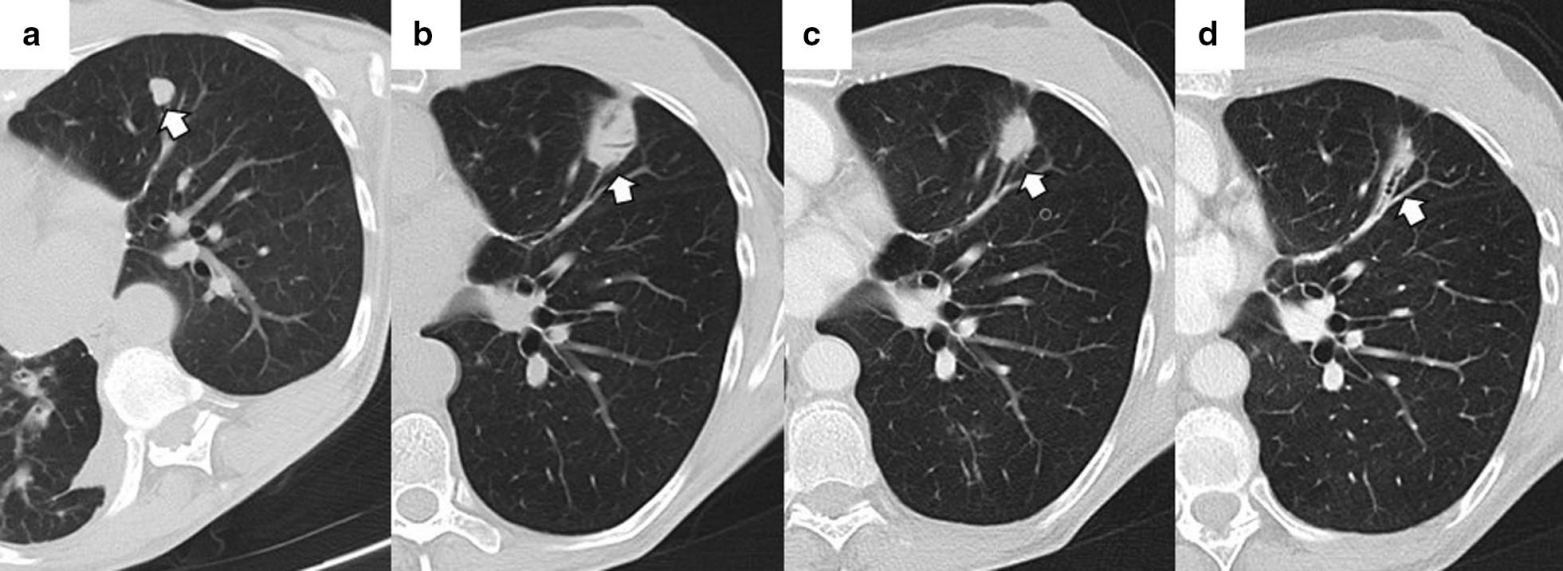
Imaging follow-up with an evolution towards fibrosis after successful treatment with image-guided percutaneous lung ablation. CT showing a metastatic lung lesion of < 2 cm due to a small cell renal carcinoma (arrow in a). The treated area has grown in size compared to the original tumor in the radiological control after one month of treatment (arrow in b). However, in the radiological follow-up performed following three (c) and six months (d) after treatment, it successively reduces its size until only an area of fibrosis remains (arrow in d)
